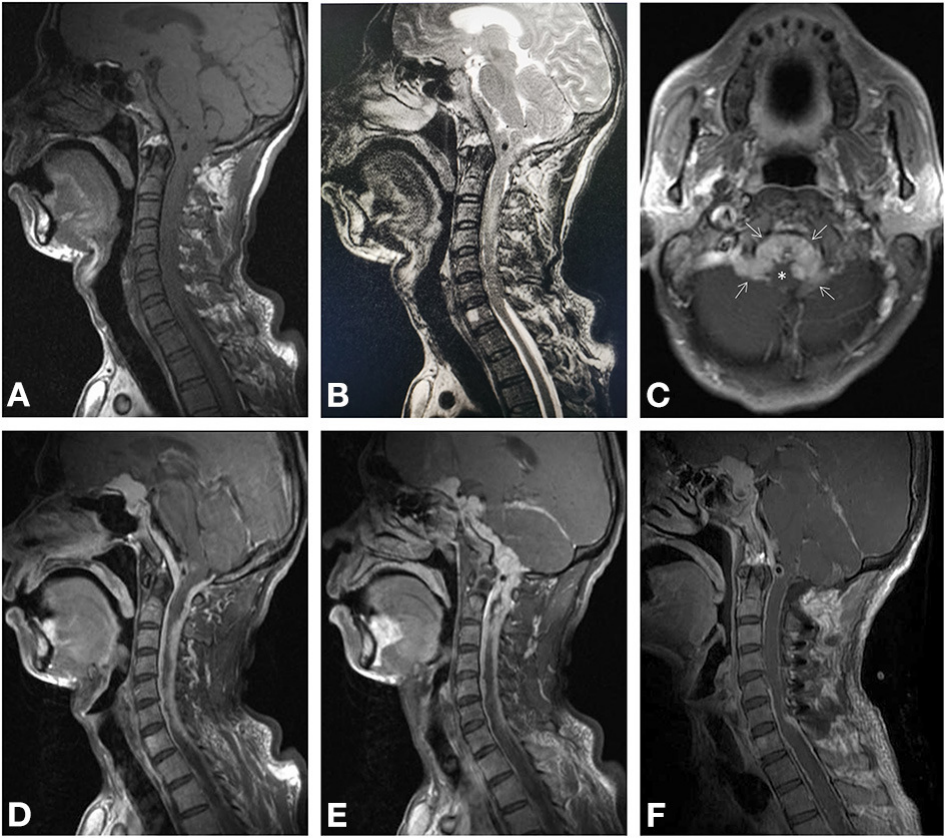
Magnetic resonance imaging. Preoperative imaging revealed isointense lesions on T1-weighted (A) and T2-weighted imaging (B), compressing the spinal cord. Axial enhanced T1-weighted imaging (C) revealed enhanced lesion located at midline premedullary cistern and lateral medullary cisterns (*arrows*), compressing the brainstem (*asterisk*). Sagittal enhanced T1-weighted imaging (D, E) showed multiple nodular lesions continuously creeping through the tentorium and meninges of sellar region, skull base, and C1–C6. Postoperative-enhanced MRI (F) proved the spinal and lower region of clivus of the tumor was totally removed.
